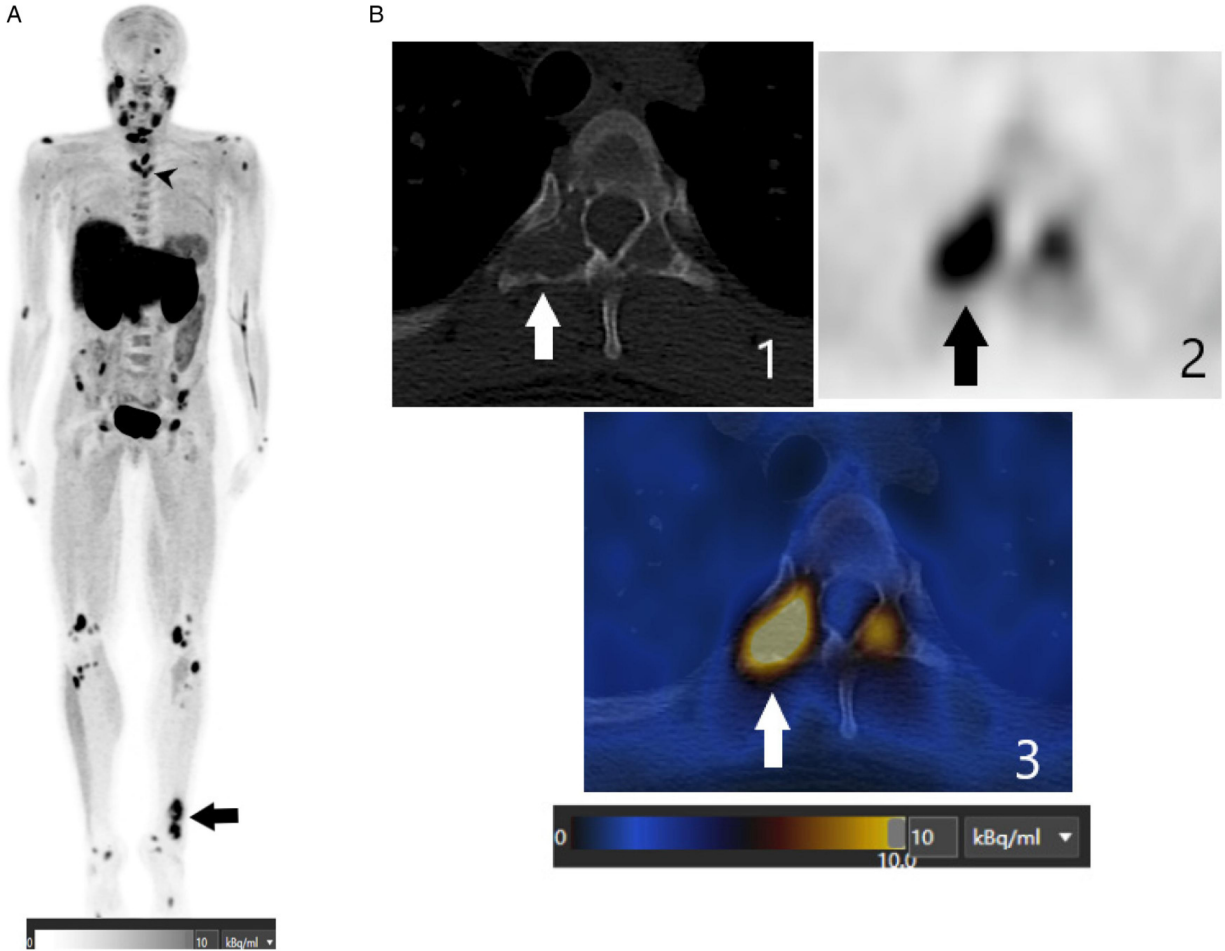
(A) This FCH PET/CT maximum intensity projection (MIP) image shows multiple abnormal foci spread throughout the skeleton, including the axial and peripheral skeleton. The black arrowhead indicates a lytic lesion in the Th4 thoracic vertebra. The black arrow indicates a lytic lesion in the left ankle. (B) These axial FCH PET/CT images (1: CT image, 2: PET image, and 3: fusion image), centered on the Th4 thoracic vertebra, show intense FCH uptake (SUVmax of 11.5) in the right transverse process, corresponding to a lytic lesion depicted by the arrows.
